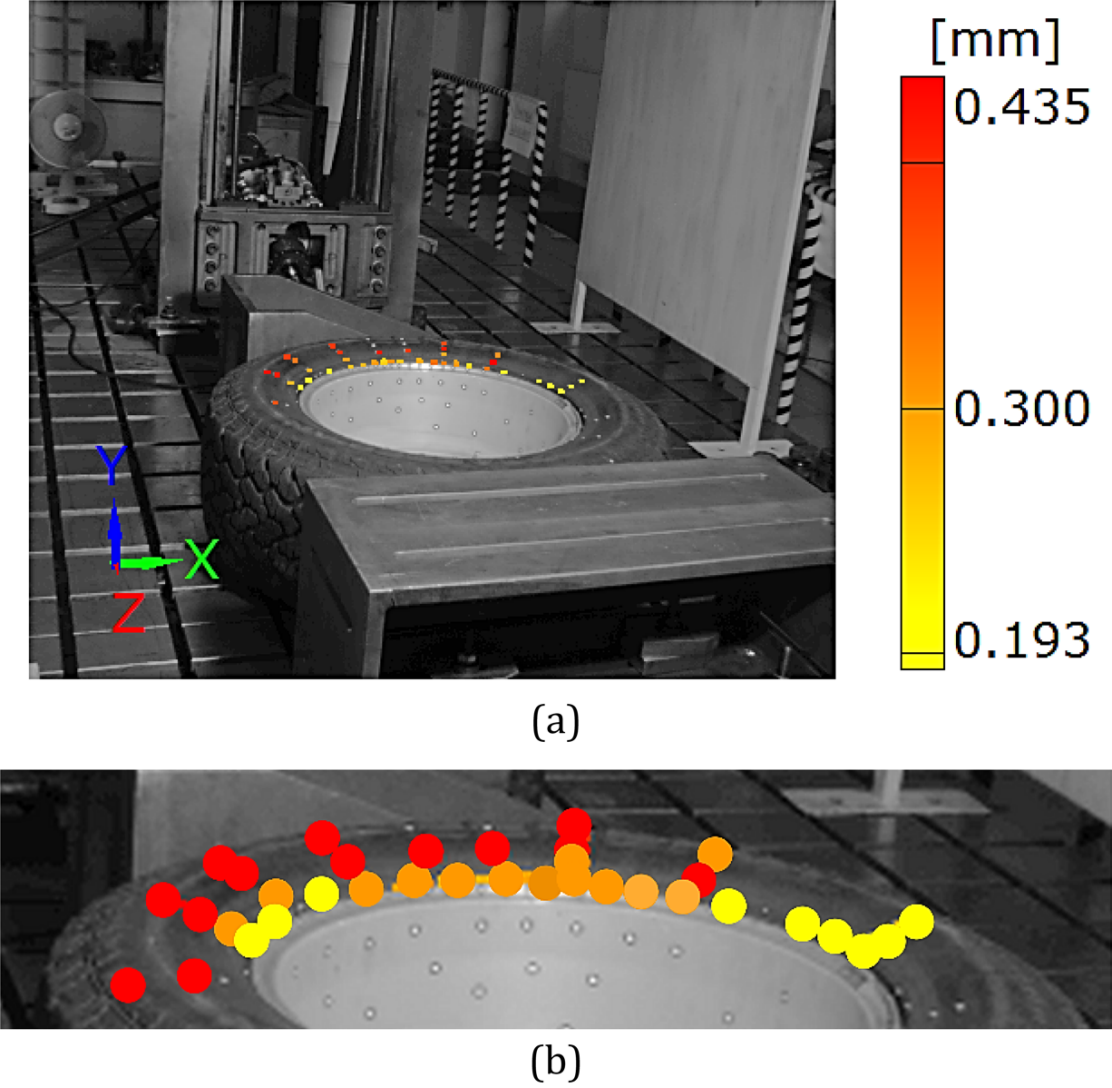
Distribution of the resultant vectors of deflection for the SUV tire after unloading: (a) general view, (b) magnified view of the tire.
